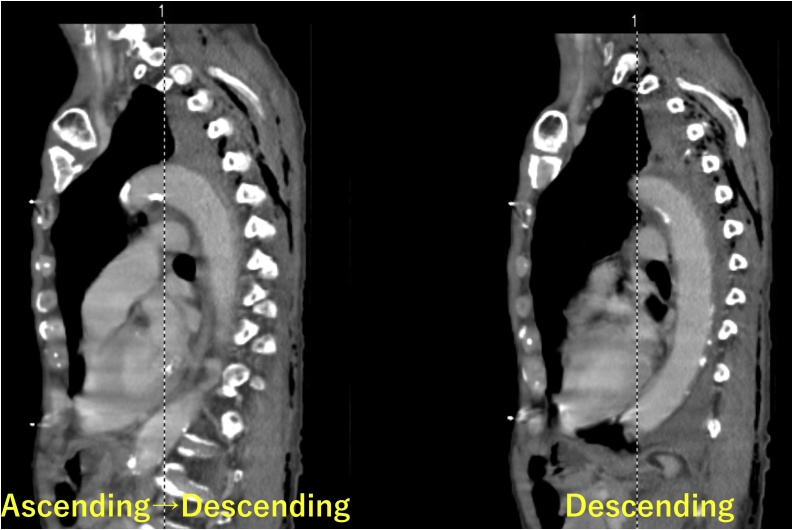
Late equilibrium phase contrasted computed tomography (CT) on admission in the local hospital. Sagittal CT cut showing no sign of aortic injury, such as extravasation, dissection, or pseudoaneurysm.
